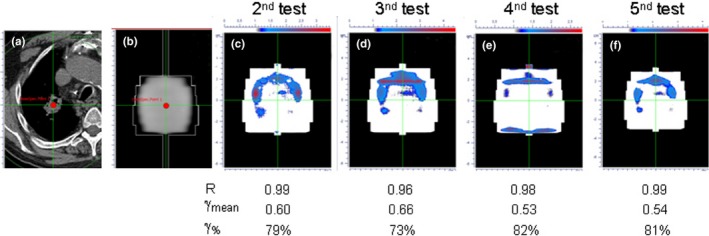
Detection of dose discrepancies in a patient treated in free‐breathing using IVD procedure. From left to right, (a) the CT scan at isocenter level; (b) the reference integrated EPID image; (c–f) the IVD tests showing a poor treatment reproducibility probably due to the residual tumor motion and a blurring dose effect.
